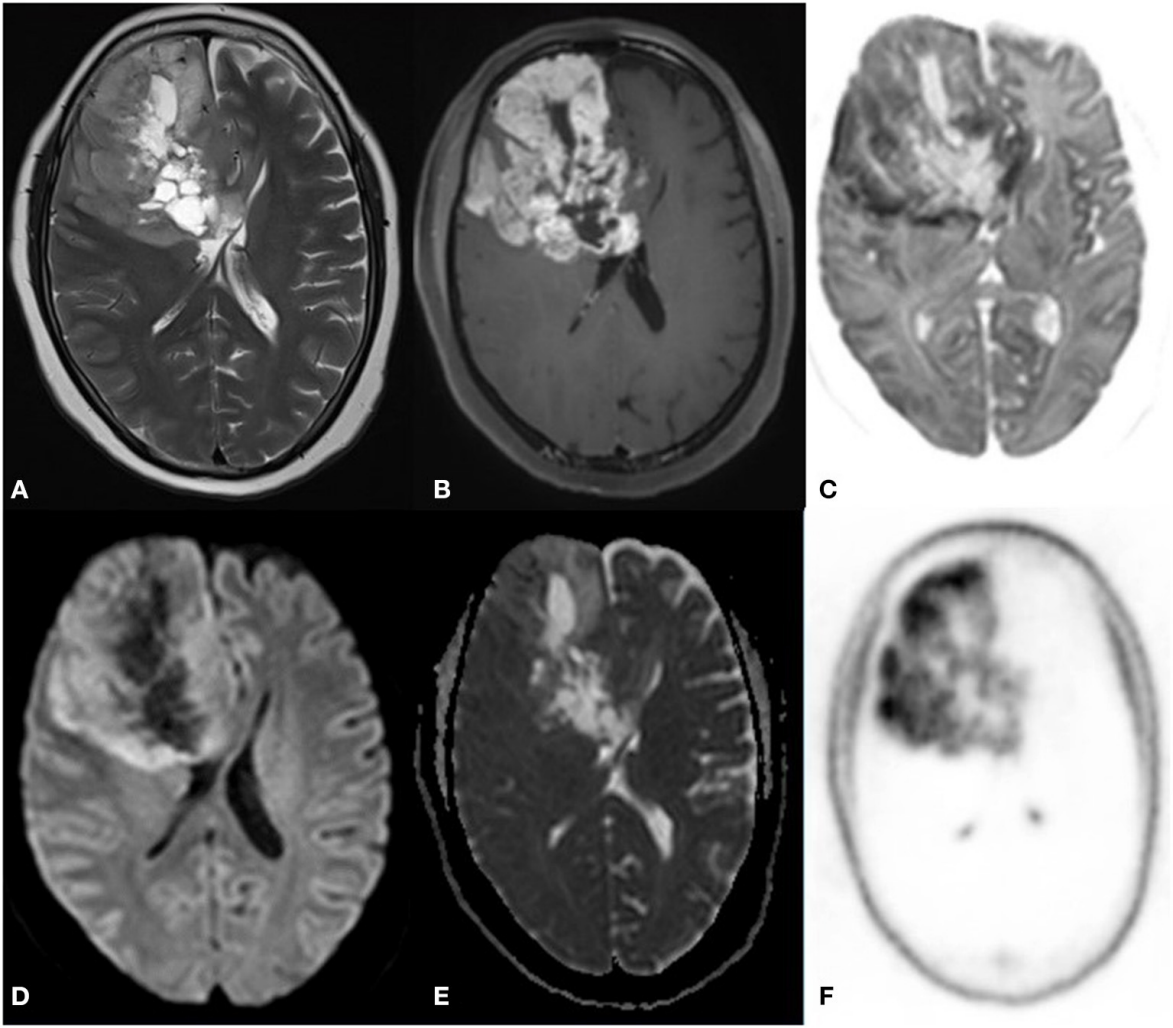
A 52 year old female with a headache for 1 year with recent onset of a seizure. MRI PET (F18 CHOLINE) axial images T2W (A) post-contrast TW (B) Inverted DWI (C) DWI b2000 (D) ADC (E) NAC PET (F) shows Ill-defined infiltrative heterogeneous lesion in the right frontal lobe with invasion to corpus callosum showing intense heterogeneous enhancement. The lesion shows DWI restriction with hypermetabolism on PET. There is a match on the inverted DWI image and the NAC PET image, which is suggestive of Glioblastoma.
